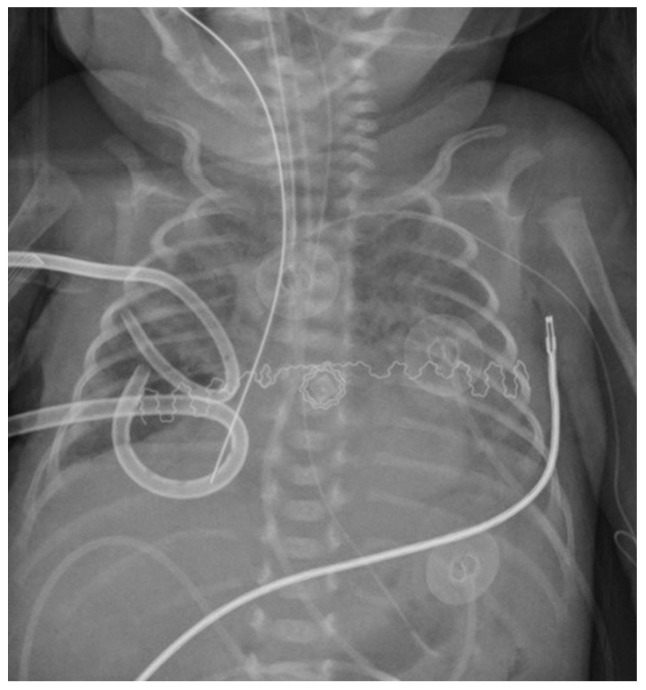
X-ray on the 17th day of life, 24 h after the occlusion. The Fogarty’s catheter in the right bronchus reduces pneumothorax and partial collapse of the right lower lobe.
